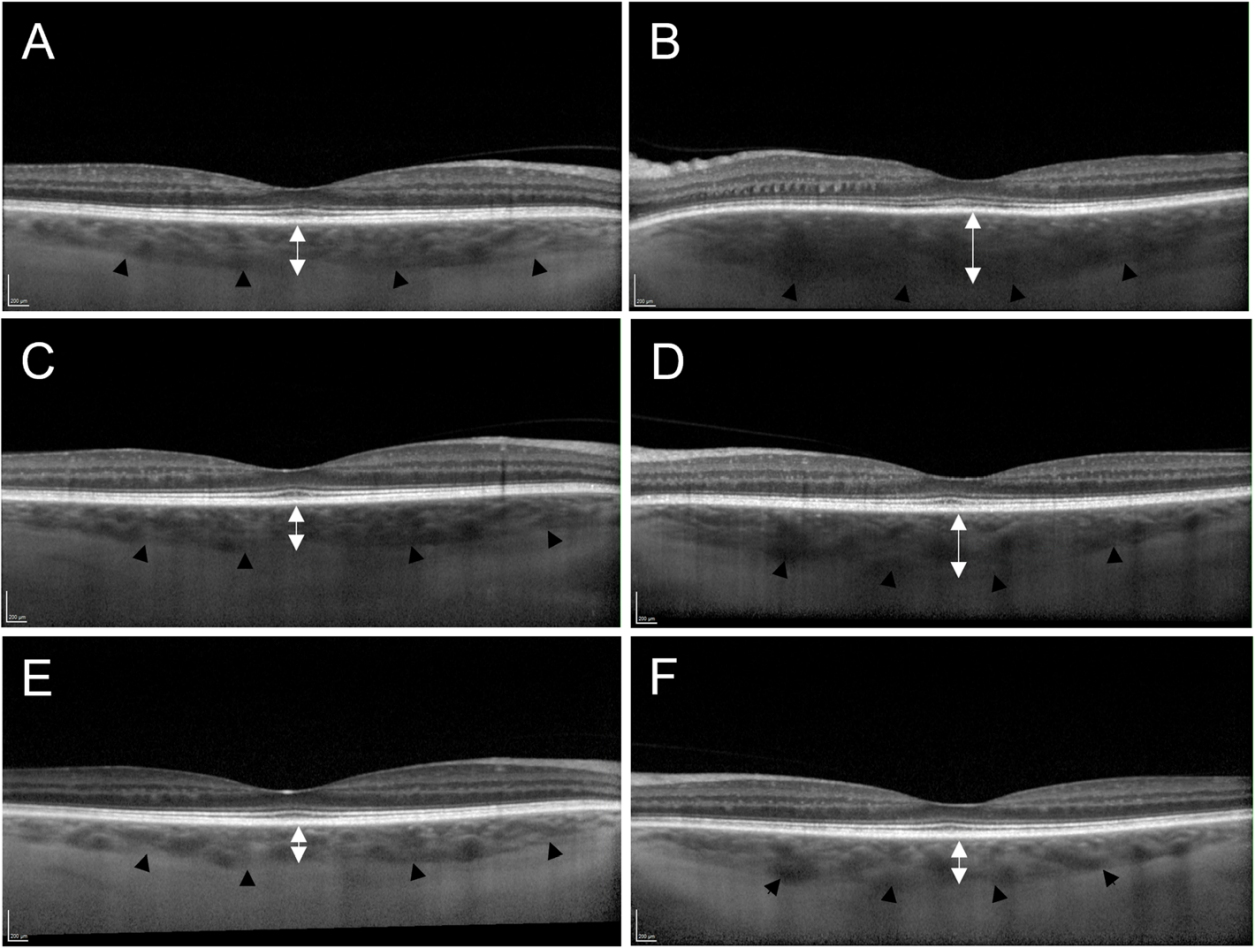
Enhanced-depth imaging optical coherence tomography (EDI-OCT) in a case of left posterior scleritis. The black arrow heads delineate the choroidal-scleral junction. (B) Spectralis EDI-OCT demonstrates left subfoveal choroidal thickening on presentation (442μm). The B-scan ultrasound showed thickening of posterior coats (2.5mm) and fluid in Tenon's capsule and optic nerve sheath (not shown). (D) Subfoveal choroidal thickness in the left eye decreased 1 week (406μm) and (F) 4 weeks (292μm) after initiation of oral prednisolone. (A,C,E) Normal subfoveal choroidal thickness in the clinically unaffected right eye of the same patient at corresponding points in time
